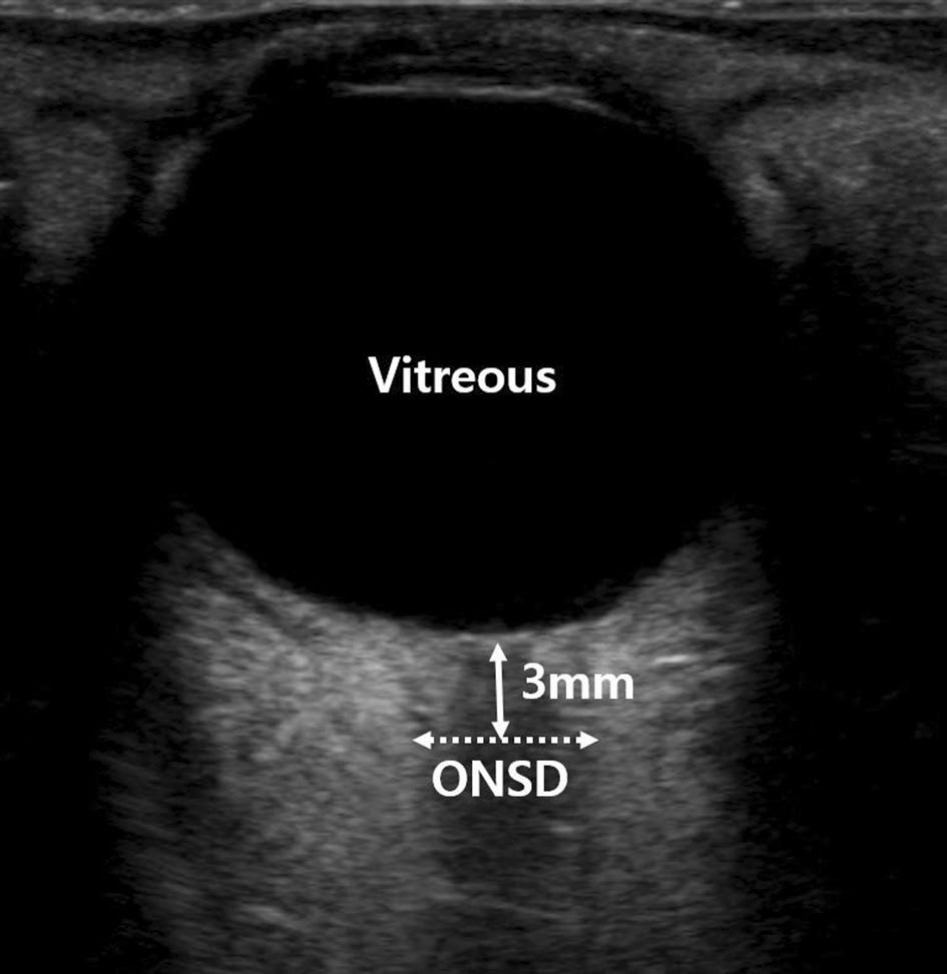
Measurement of optic nerve sheath diameter (ONSD) by ultrasonography. The outer diameter of the optic nerve sheath was measured 3 mm behind the optic disc.
